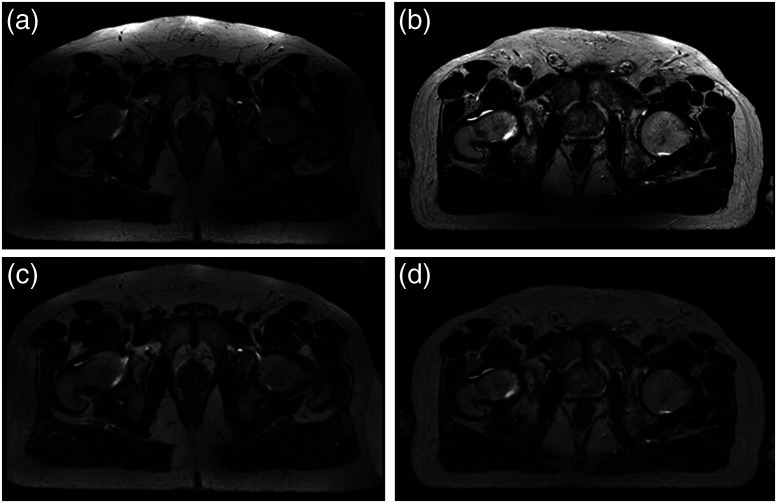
(a) and (b) represent the MR images of the same anatomical slice in two different patients obtained within the same scanner, where inhomogeneity and intensity non-standardness are very well demonstrated. (c) and (d) show the same slices after bias field correction.
